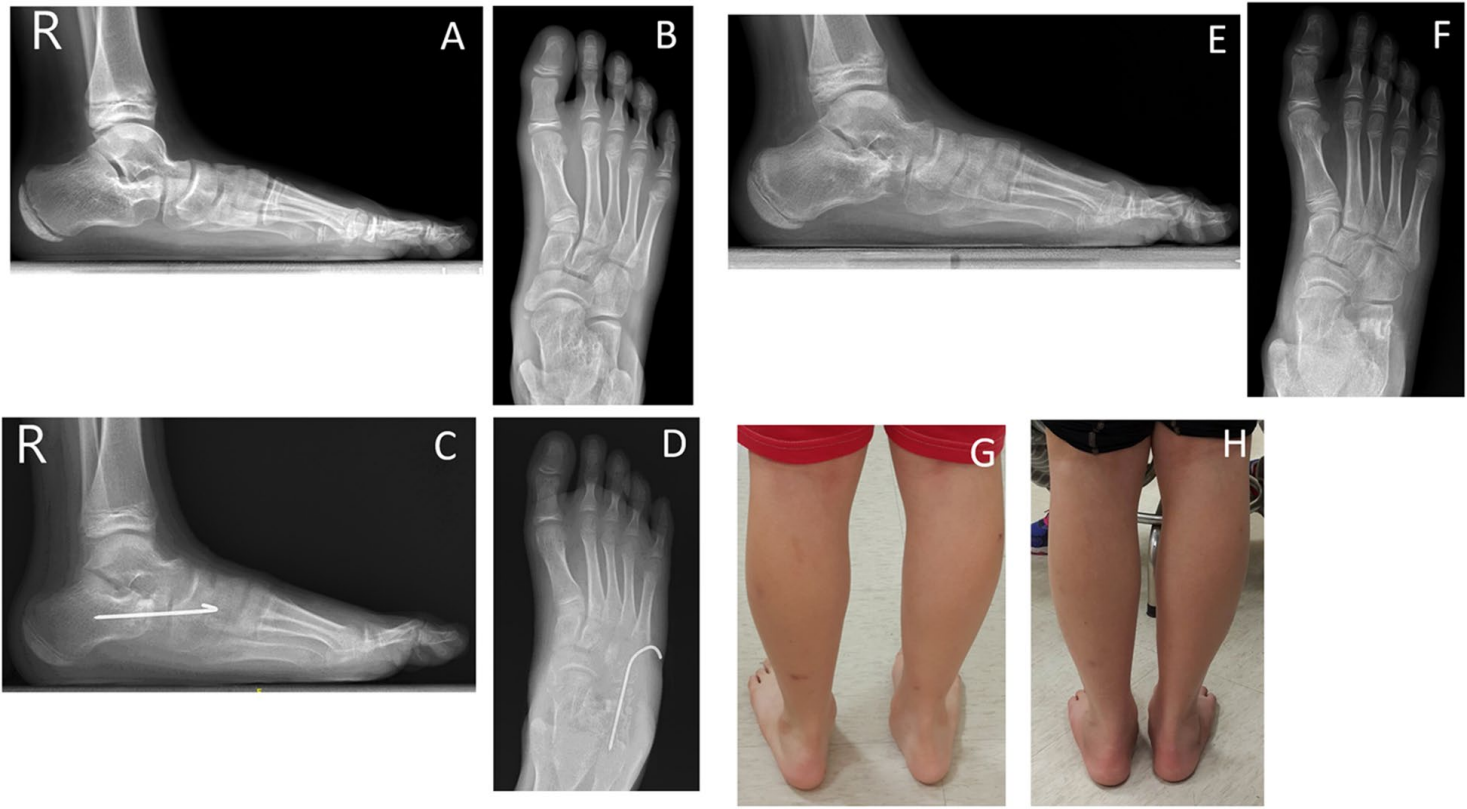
A and B A 13-year-old boy with symptomatic right flexible flatfoot. C and D One-month postoperative radiograph showing correction of the calcaneal pitch and the anteroposterior and lateral Meary angles with mild calcaneocuboid (CC) joint subluxation. E and F Three-month postoperative radiograph showed full union and no graft sinking or graft collapse at the lengthening site. The CC subluxation showed improvement and the clinical outcome was excellent. G Preoperative and H 3-month postoperative clinical photos
